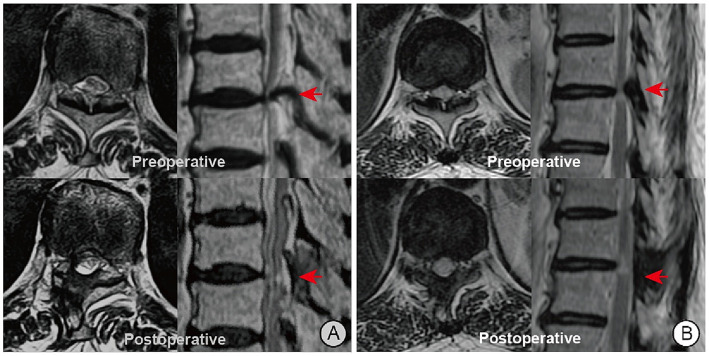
Preoperative and postoperative MRI images of representative cases: fused type (A) and non-fused type (B). Spinal cord compression status before and after surgical intervention (red arrows).
